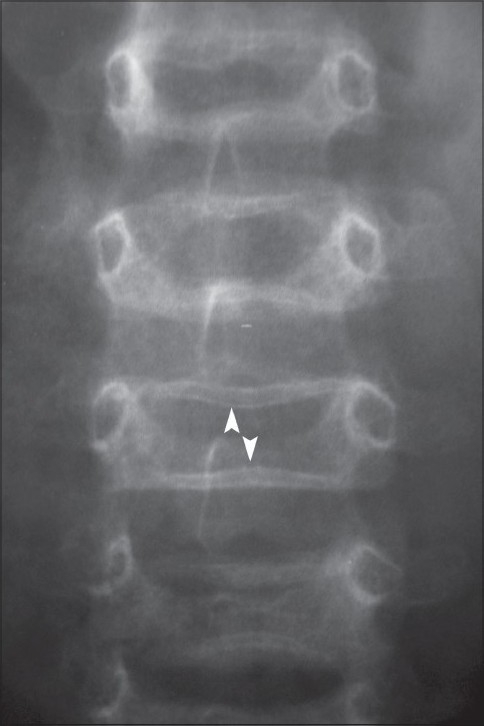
Frontal radiograph of the lumbar spine showing metaphyseal bands (white arrow heads)
